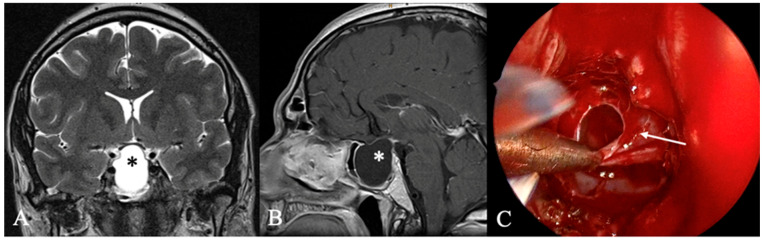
A 67-year-old male patient with a diagnosis of Rathkes cleft cyst. (A) T2-weighted and (B) contrast-enhanced MRI of a typical Rathke cleft cyst (*). Typical appearance with high T2 and low T1 signal and thin contrast-enhancing capsule. (C) Intraoperatively, these cysts appear benign, fluid-filled lesions with a favorable prognosis and a low recurrence rate. The arrow indicates the cyst wall.
